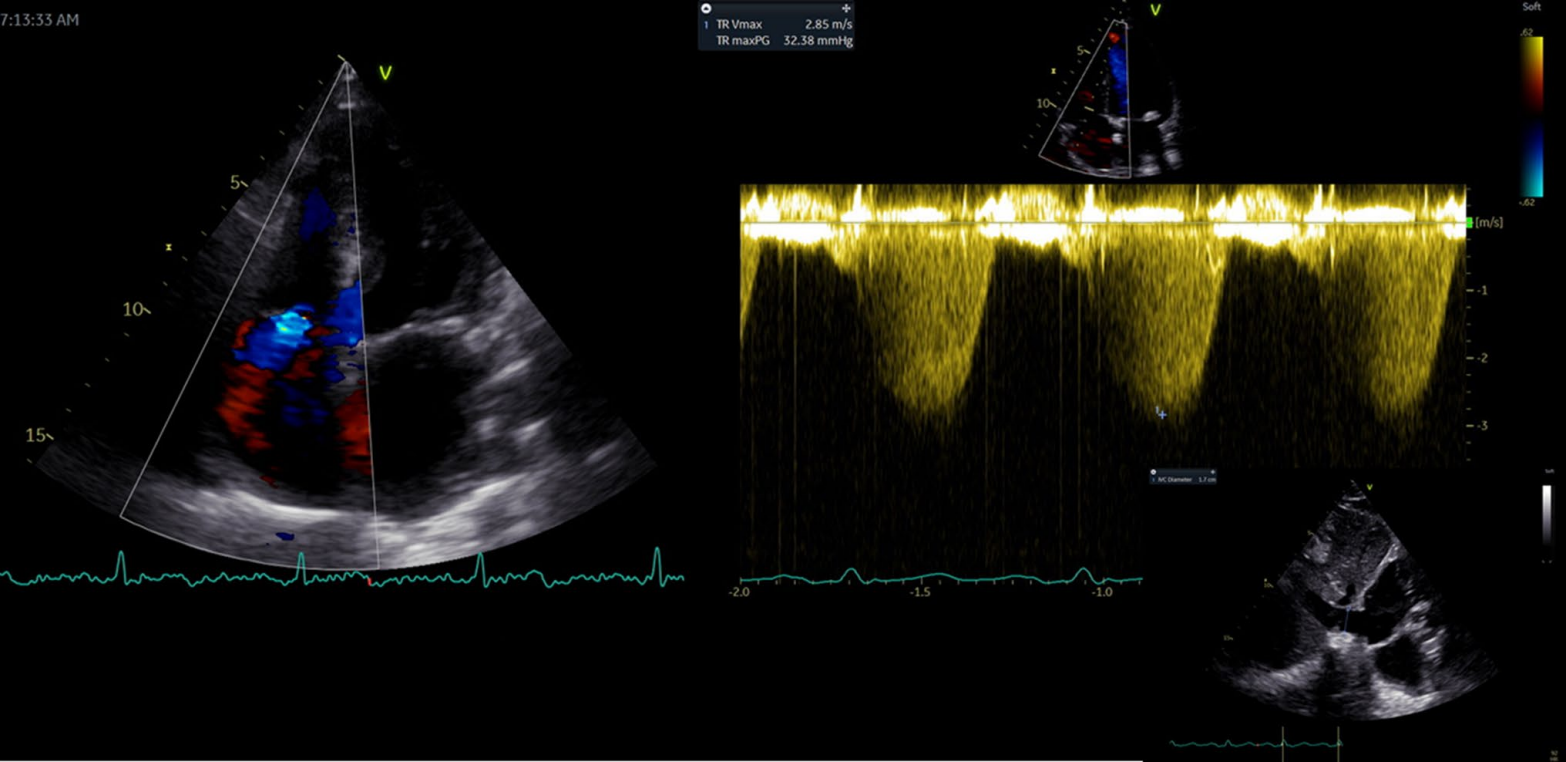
Echocardiographic pulmonary artery systolic pressure (PAP_systolic_) obtained by adding gradient corresponding with tricuspid regurgitation peak velocity to estimated right atrial pressure obtained from inferior vena cava size and collapse. Pulmonary artery mean pressure was calculated as 0.6 × PAP_systolic_ + 2
